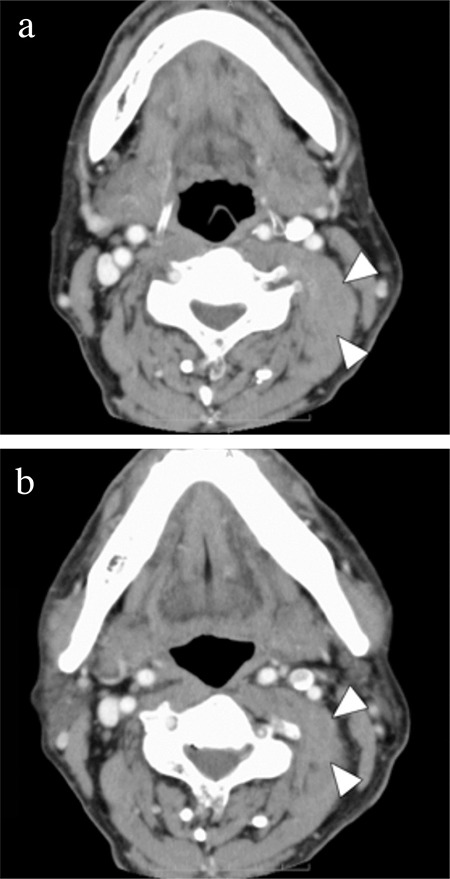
Contrast enhanced-computed tomography findings of left posterior neck mass (a, b). Contrast enhanced-computed tomography scan reveals a large soft tissue mass in the left posterior neck, involving both the anterior and medius scalene muscles (white arrow head).
